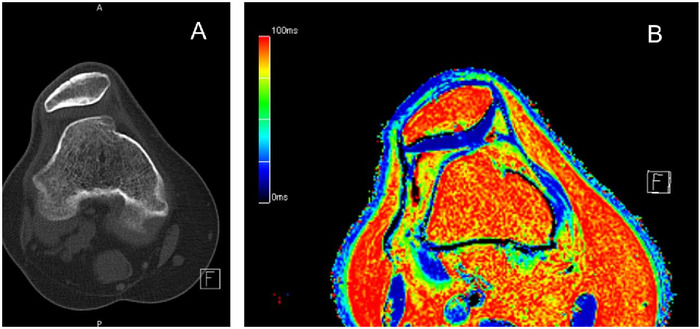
(A) Computed tomography scan of the right knee shows lateral patellar subluxation and trochlear Dejour type D dysplasia. (B) T2 mapping magnetic resonance imaging of the right knee shows minimal articular cartilage damage but continued lateral patellar subluxation.
